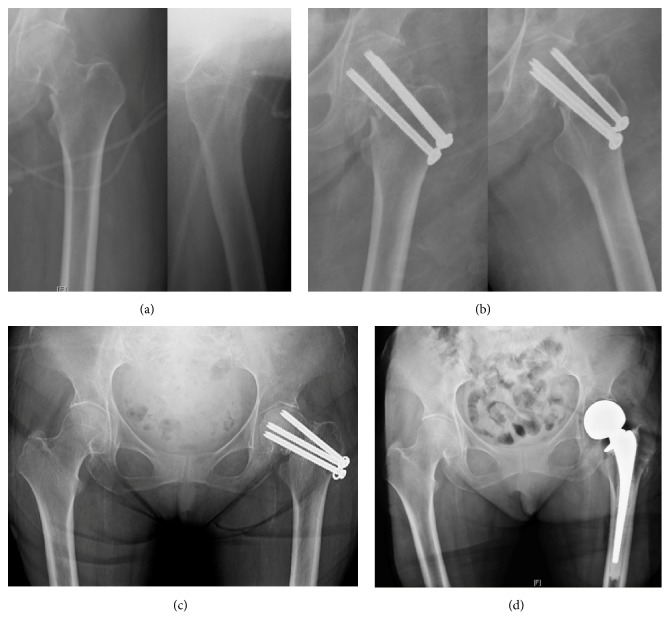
(a) A 61-year-old female sustained a syncopal fall at home resulting in a displaced left transcervical femoral neck fracture. She has a history of multiple medical comorbidities including end-stage renal disease on hemodialysis, diabetes mellitus, and hypertension. She subsequently went into PEA arrest twice during transport to the hospital. She was intubated and sedated on arrival. She remained critically injured in the Intensive Care Unit and was deemed an extremely high risk for surgery; therefore surgical intervention was delayed until the patient was medically stable. On hospital day 8, the patient was cleared by the medical service for surgical intervention of her displaced femoral neck fracture, and after extensive conversation with the patient and her family, consent was obtained and surgery was pursued with the plan for hemiarthroplasty given her fracture displacement and delayed presentation to the OR. After induction of anesthesia, the patient was deemed too instable to undergo a hemiarthroplasty, so conversion to closed reduction with percutaneous cannulated screws was chosen, aware of the chance of failure, but choosing this given her medical instability and high risk (b). She was followed in the clinic and over the following 7 months continued to have groin pain with ambulation and radiographic signs of hardware failure and nonunion (c). During this time, she had extensive medical optimization in anticipation of revision surgery. When deemed medically optimized and cleared for surgery, after discussion with the patient and her family, she was brought back to the OR for hardware removal and left hip hemiarthroplasty (d). She tolerated the procedure without complications and is ambulating with minimal pain postoperatively.
